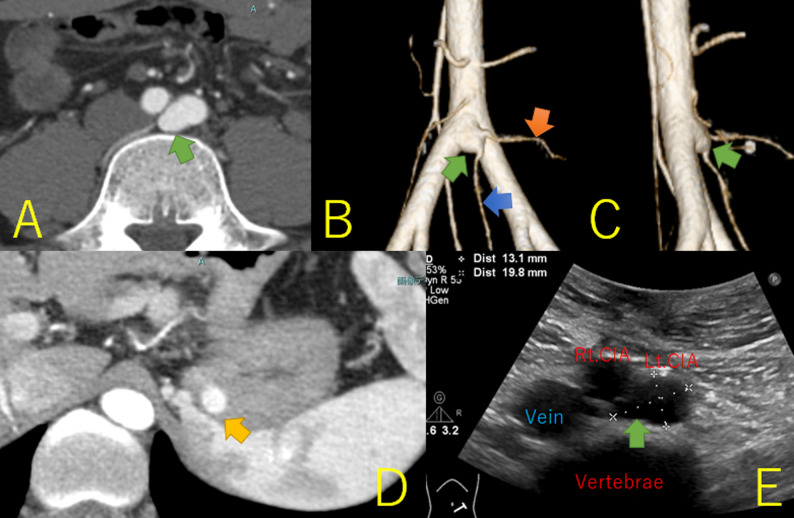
Preoperative findings. A Contrast-enhanced computed tomography (CT) revealing a saccular aneurysm (green arrow) measuring 20 mm in diameter at the origin of left common iliac artery(CIA) or the bifurcation of the terminal aorta. B Anterior-view three-dimensional CT image. The median sacral artery (blue arrow) and the left fourth lumbar artery (orange arrow) generated from the aneurysm (green arrow). C Left-sided three-dimensional CT image. The aneurysm (green arrow) was located at the L4/L5 level and protruded to the front of the lumbar vertebrae. D Gastric varix (orange arrow) measuring 8 mm in diameter E Ultrasonography showed the aneurysm (green arrow) measuring 13.1 × 19.8 mm in diameter at the origin of the left CIA
